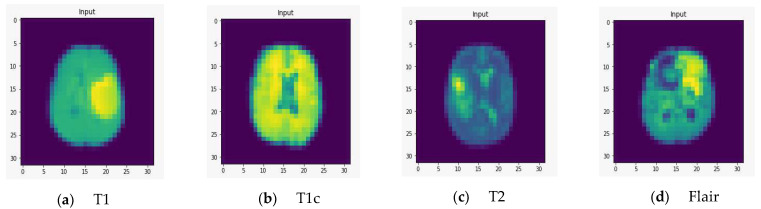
Rendering of T1, T1c, T2, and FLAIR at a resolution of 32 × 32 × 32.
